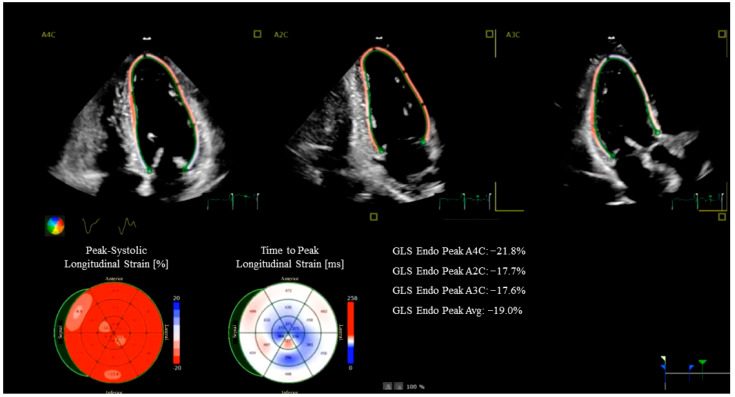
Strain Echocardiography in a coronary artery bypass grafting patient. The images show a reduced global longitudinal strain (GLS) in the anterior septum and inferior wall.
